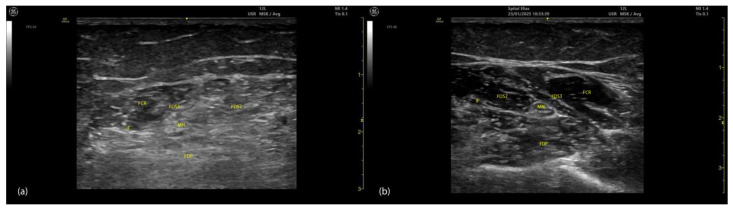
Comparison of ultrasound characteristics in spastic and normal muscles. (a) ultrasound image of spastic muscles (e.g., FDS3 and FDS2) showing altered echogenicity, disrupted intermuscular fascia (IF), and structural changes near the median nerve (MN) and adjacent FDP muscle; (b) ultrasound image of the same muscles in a normal state, demonstrating uniform echotexture, intact IF, and clear differentiation between muscle layers and the MN; FDS3—flexor digitorum superficialis digit 3; FDS2—flexor digitorum superficialis digit 2; MN—median nerve; IMF—intramuscular fascia; FDP—flexor digitorum profundus; FCR—flexor carpi radialis.
